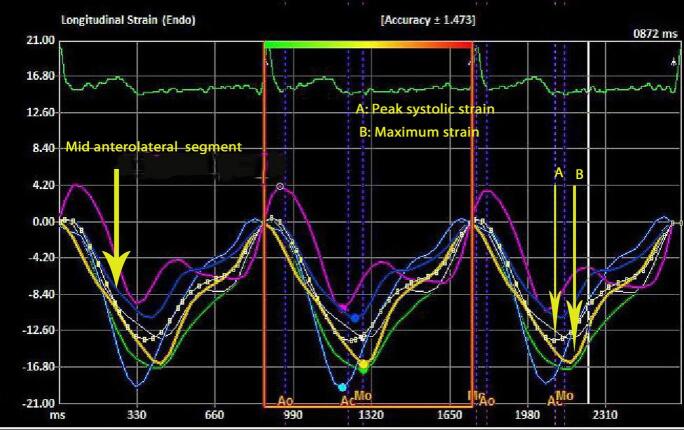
Longitudinal speckle-tracking echocardiography in apical four chamber view displays strain curves in inferoseptal and anterolateral LV myocardial walls by dividing them into three segments consist of basal, mid and apical segments. The PSI is calculated by this formula: (maximum negative strain in cardiac cycle - peak negative systolic strain)/ (maximum negative strain in cardiac cycle) x 100. Yellow vertical arrows display peak negative systolic strain and maximum negative strain in cardiac cycle in mid anterolateral myocardial segment (LV: Left ventricle; PSI: Post systolic index). (For interpretation of the references to colour in this figure legend, the reader is referred to the web version of this article.)
